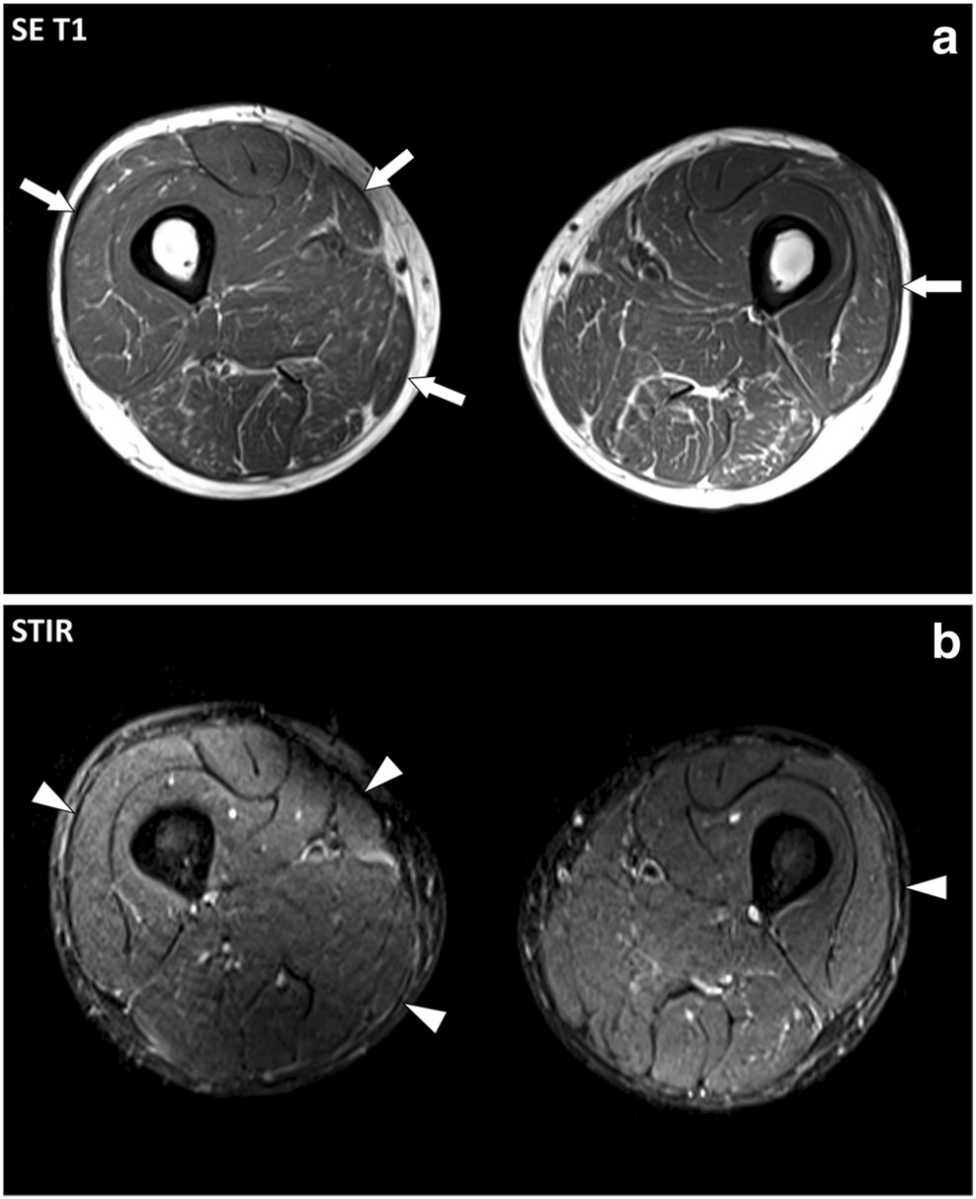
Axial (a) SE T1-weighted and (b) STIR images of the thighs of a 52-year-old male with eosinophilic fasciitis treated for a year. MRI demonstrates thickening (arrows) of the deep peripheral fasciae with low signal intensity on STIR images (arrowheads) suggesting fibrosis
